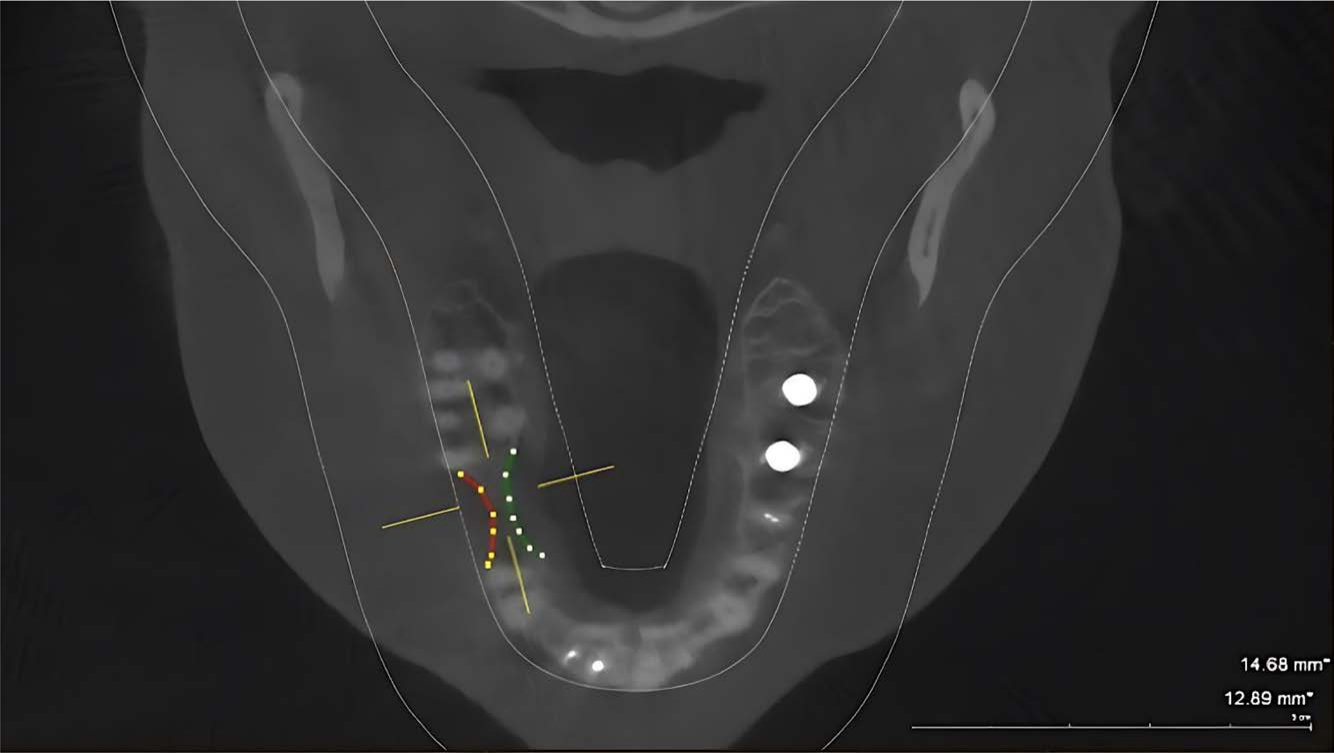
CBCT for Siebert’s classification bone defects at mandibular right class I for female patient. CBCT = cone beam computed tomography.
